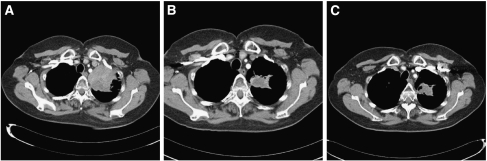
Partial response in a patient with inoperable non-small-cell lung cancer receiving sunitinib 37.5 mg per day and irinotecan. CT scans are shown at baseline (A), after 7 weeks (B) and 6 months (C) on treatment.
